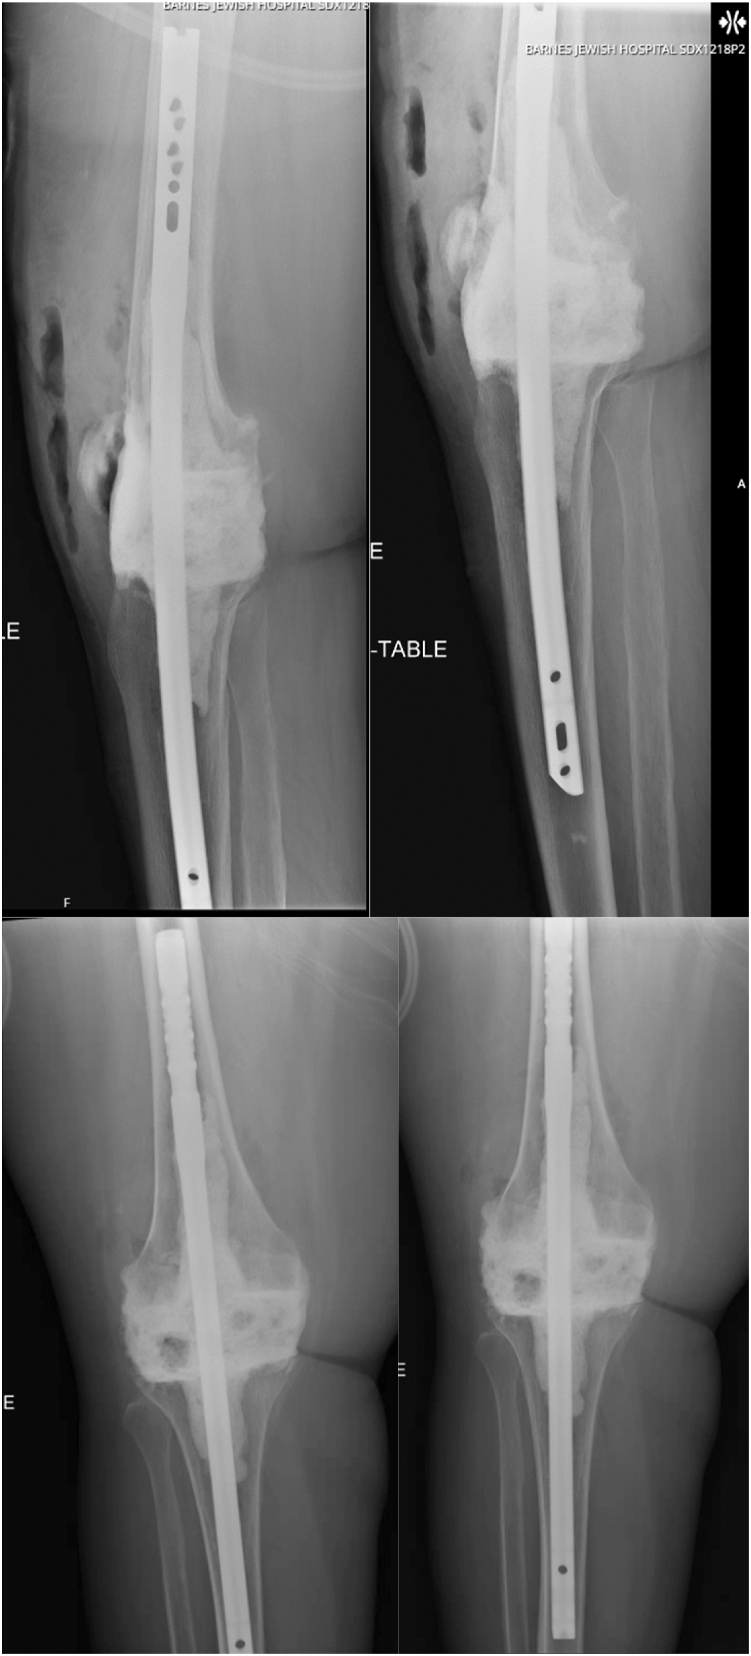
Standard anteroposterior and lateral radiographs capturing the proximal and distal extent of the nail are obtained postoperatively.
